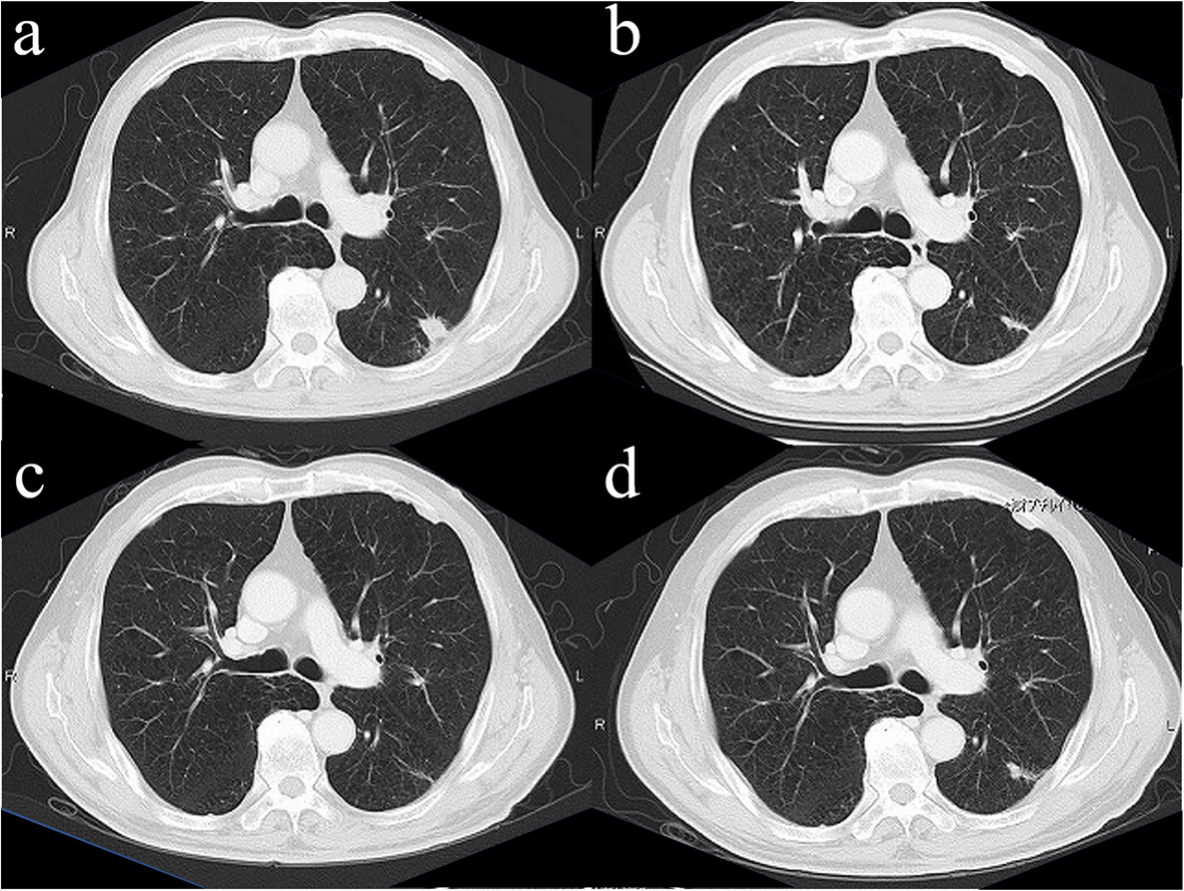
Computed tomography (CT). a Chest CT showed a well-circumscribed, 1.6-cm mass in the S6 segment of the left lower lobe. b CT performed before chemotherapy and 2 months after left adrenalectomy showed spontaneous regression of lung lesions. c After chemotherapy, the lung lesions became linear and nearly disappeared. d Lung lesions re-enlarged 11 months after adrenalectomy
